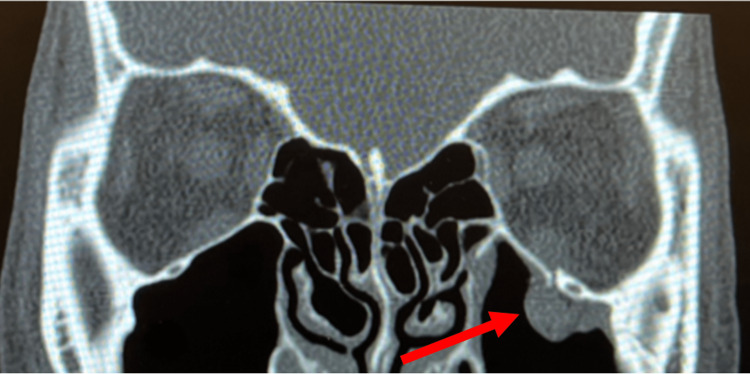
A coronal view of the non-contrast CT (soft tissue window) showing a right-sided orbital floor trapdoor fracture with associated orbital soft tissue entrapment in the fracture (red arrow).
